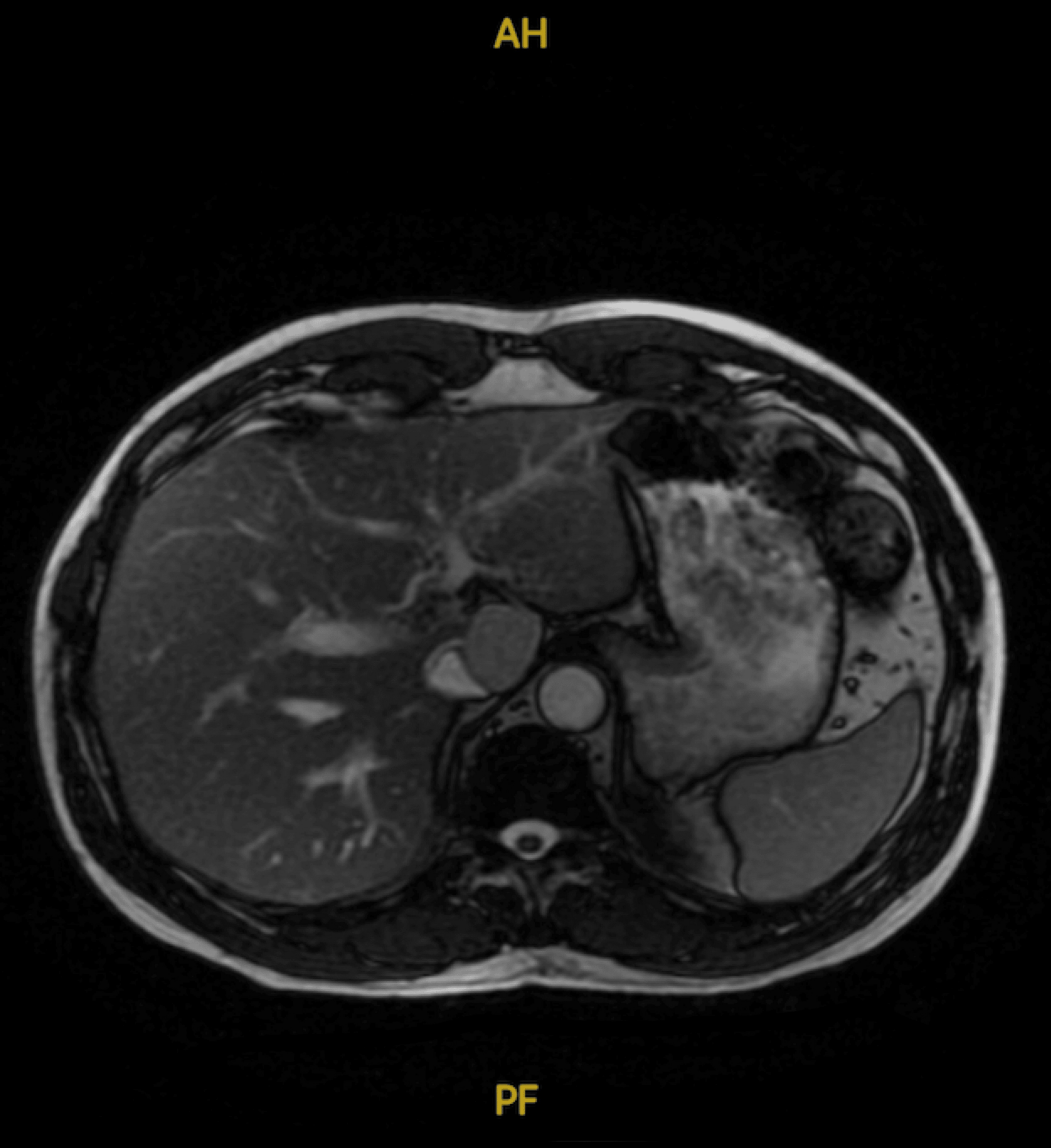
Liver MRI: smooth, regular appearance, with uniform signal intensity, normal vascular structures, and no signs of abnormalities, such as lesions, fatty infiltration, or biliary obstruction. Gallbladder wall: 2-3 mm.
